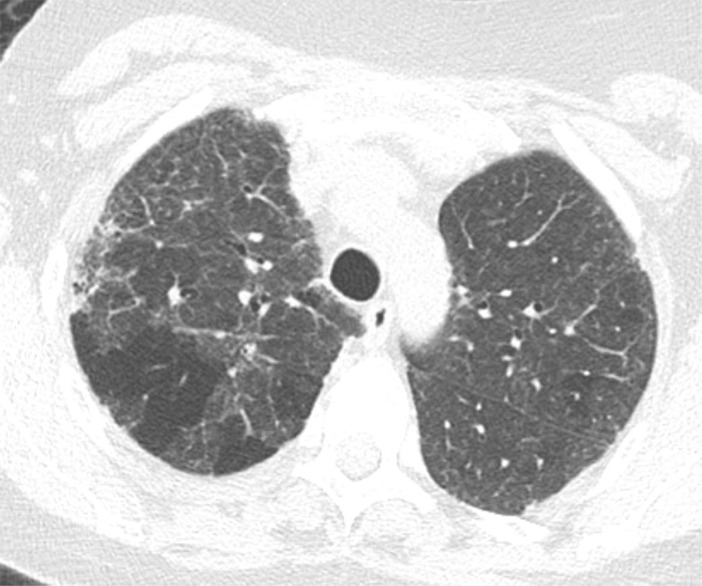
Fibrotic hypersensitivity pneumonitis. (a–c) Axial inspiratory CT scans demonstrate substantial ground-glass abnormality, mild centrilobular nodularity, and moderate mosaic attenuation, in addition to peribronchovascular and lower lung–predominant fibrosis. (d–f) Axial expiratory CT images confirm air trapping as the cause of mosaic attenuation. The degree of ground-glass abnormality, the degree of air trapping, and the peribronchovascular predominance of fibrosis would result in this case being categorized as most consistent with a nonidiopathic pulmonary fibrosis diagnosis.
